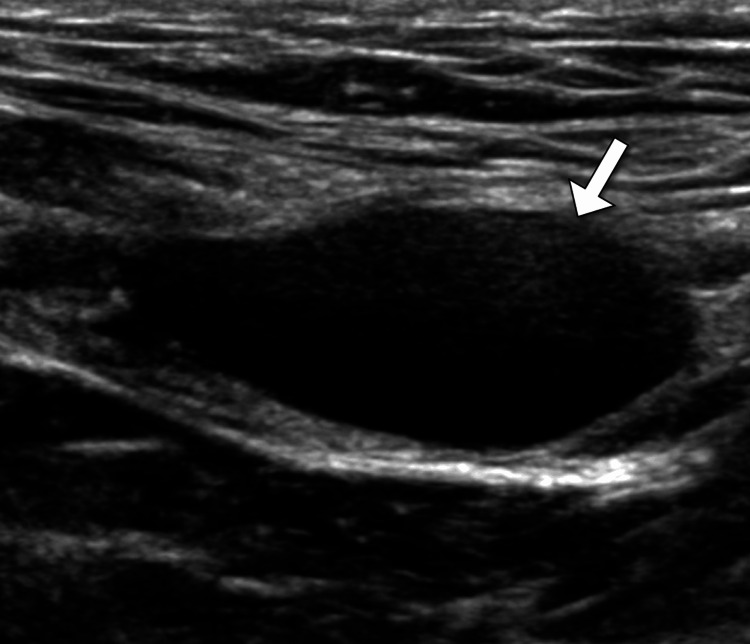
Ultrasound finding of the swelling Ultrasound examination revealed a well-defined anechoic structure without internal components, consistent with a cyst.
